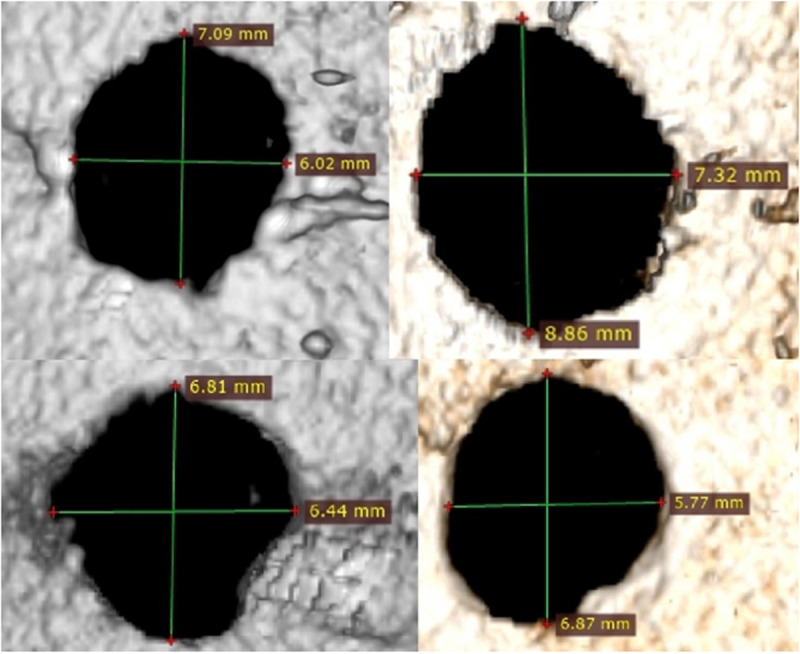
Bone entrance wound damage in 3D CT reconstruction. From upper left, clockwise – H&N Baracuda, HollowPoint, Apollo, Spitzkugel.
